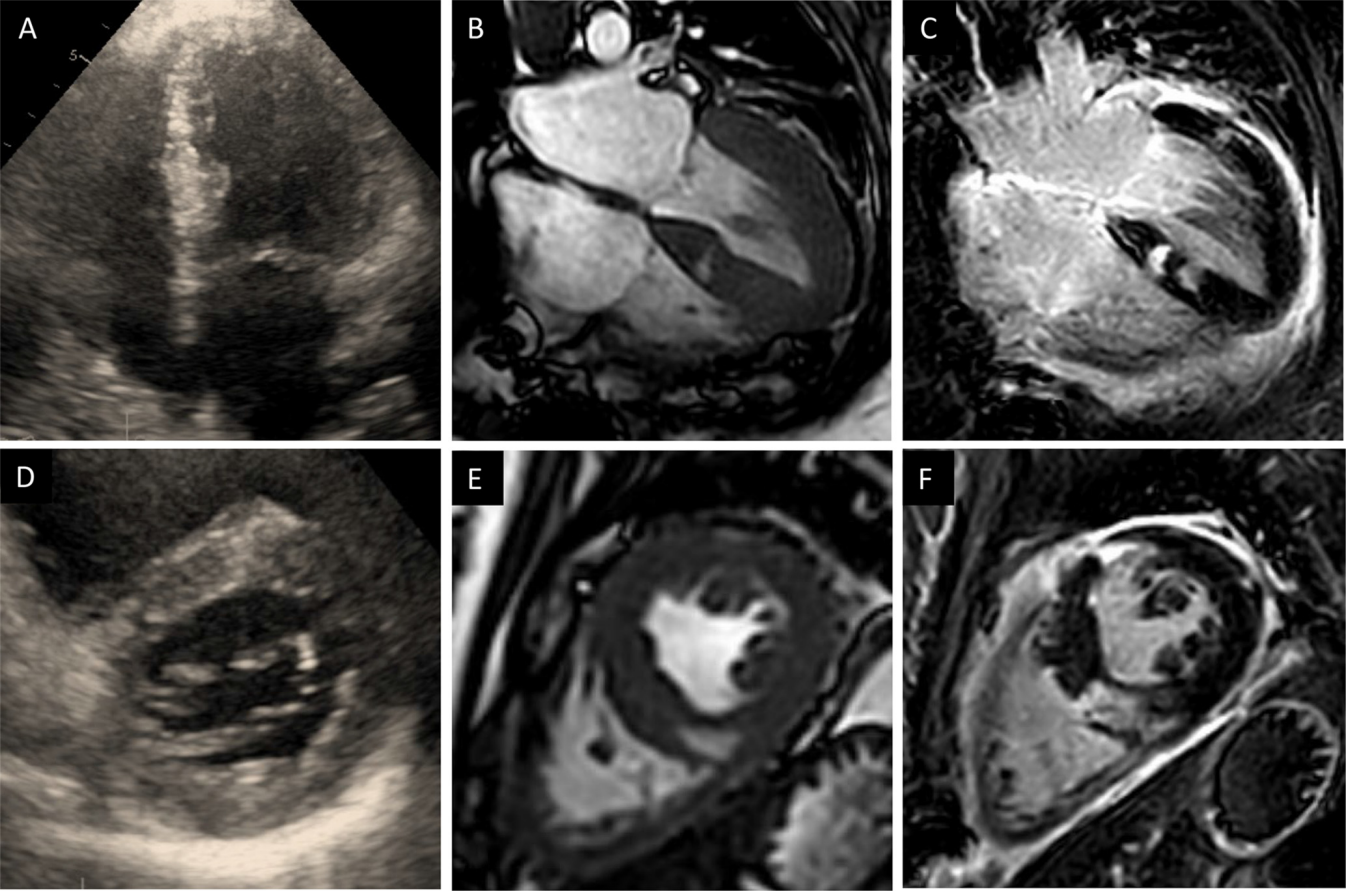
(A–C), Four-chamber transthoracic echocardiography (A) and Cardiac magnetic resonance (CMR) images (B) reveal concentric hypertrophy with moderately impaired left ventricular function and multiple cystic structures in the septal, mediolateral and anterior parts of the left ventricle. (C) Late gadolinium enhancement (LGE) demonstrated high signal intensity of the cystic lesions indicating fibrotic tissue (D–F), Short axes of the corresponding sequences: transthoracic echocardiography (D), Cine-CMR (E) and LGE (F).
